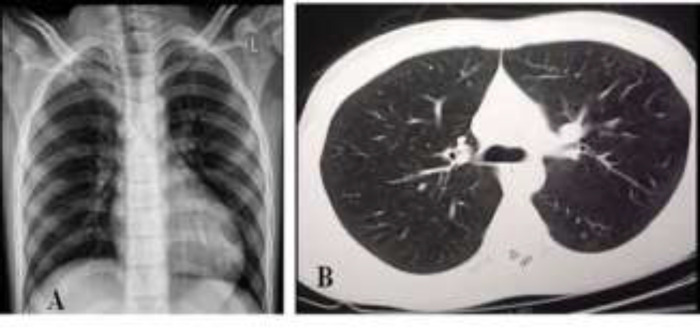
A, B: normal CXR and lung CT
